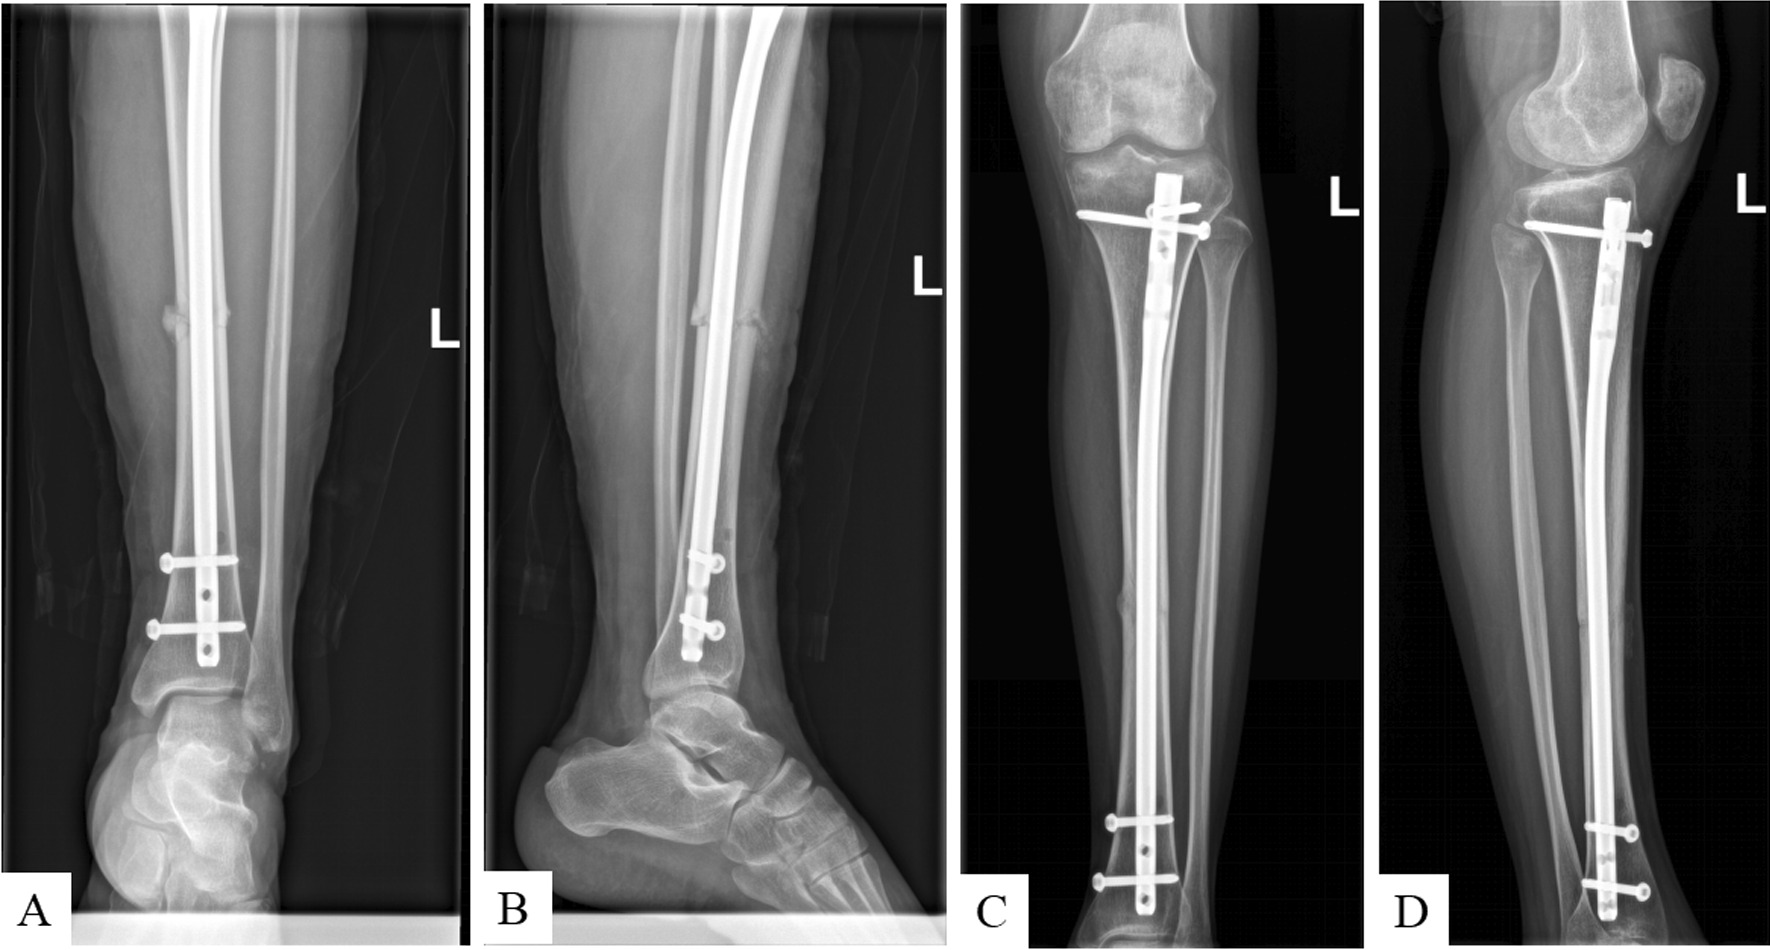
Postoperative X-rays in *G*_M_. A and B were 1 day postoperative X-rays. A perfectly fracture reduction was achieved. B Bone grafting shadow was visible. C and D were 6 months postoperative X-rays. The fracture was healing well
